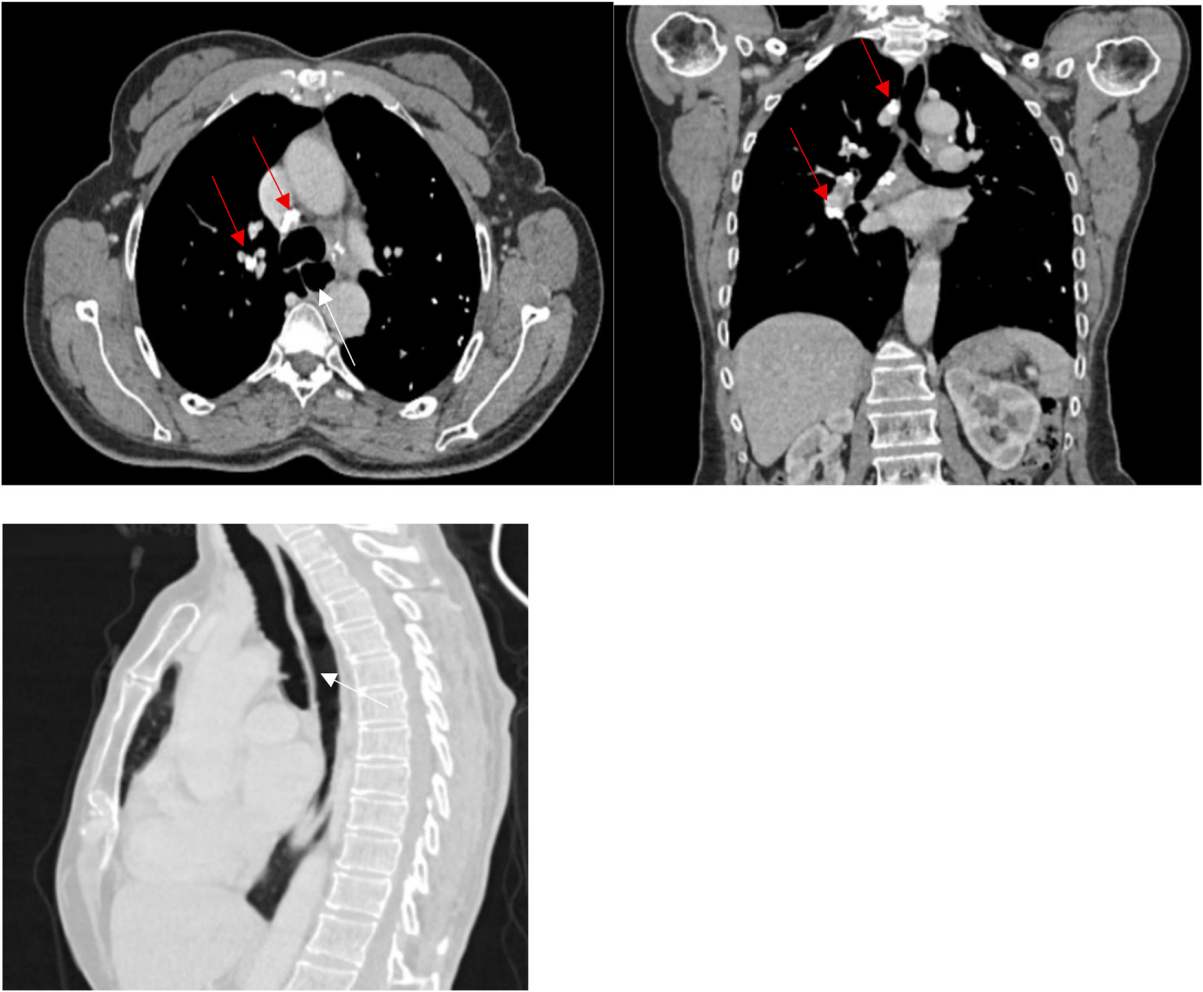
Axial and coronal CT images (mediastinal window) and sagittal CT image (parenchymal window) demonstrating mediastinal and hilar lymph node calcifications (red arrow) and esophageal dilation (white arrow).
